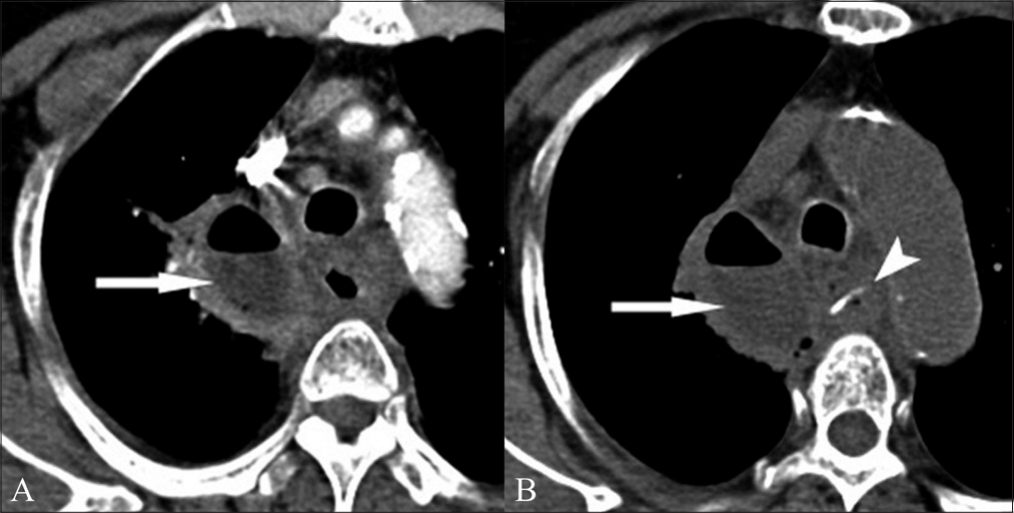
Contrast-enhanced (A) and noncontrast (B) axial CT scans show a large mediastinal abscess (arrows in both A and B) in the right paratracheal region, with associated stranding of the surrounding mediastinal fat. The esophageal wall is thickened. A linear FB (arrowhead in B) is seen within the lumen of the esophagus at the level of the arch of the esophagus
